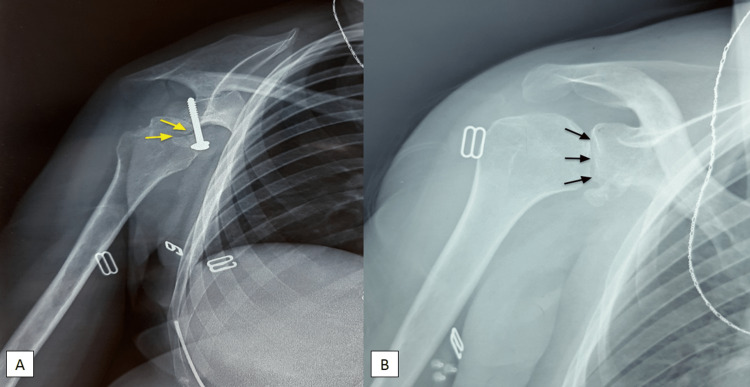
(A) Frontal X-ray showing pseudarthrosis of the coracoid bone block after four months of the Latarjet procedure (yellow arrows) with subluxation of the humeral head. (B) Frontal X-ray one month after removal of the screw showing a glenohumeral joint in place (black arrows).
